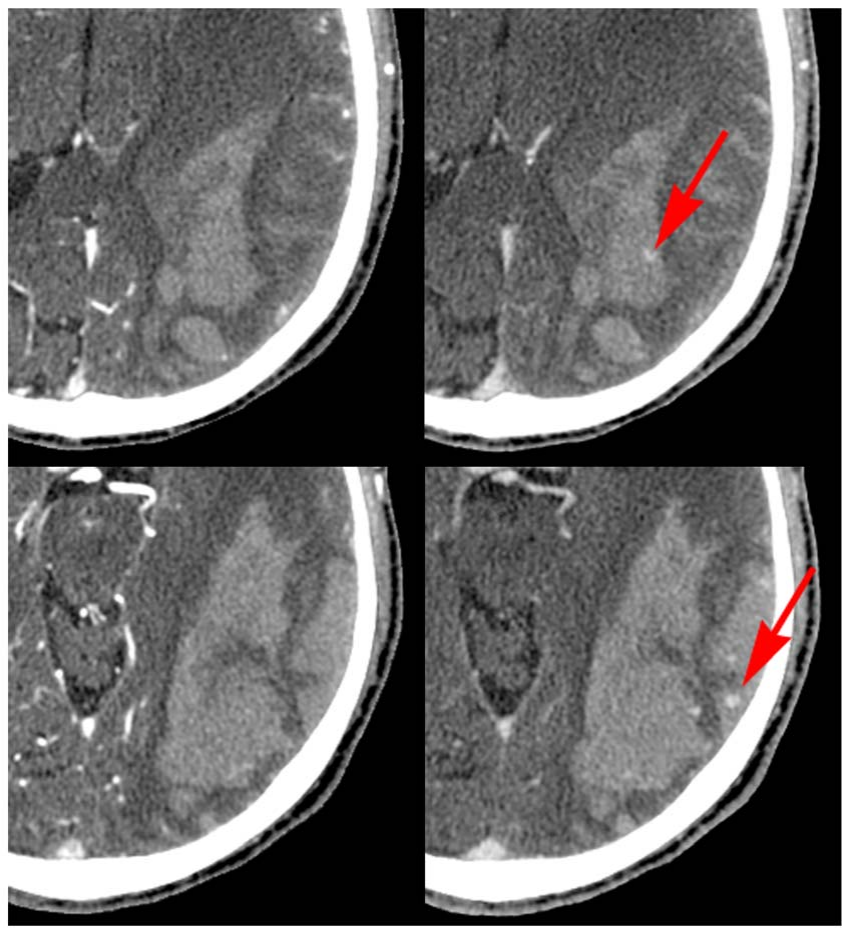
Example of active bleeding into a hematoma. 88 year old male with hypertension and acute stroke-like symptoms. CTA with delayed phase imaging shows a large hematoma in a left temporal/parietal location. Two axial levels are presented; on the left, images are from the initial phase imaging, on the right from the delayed phase. The red arrows indicate development of active extravasation seen only on the delayed views. The size of the hematoma was much larger than 80 ml and surgery was not performed, the presence of active bleeding did not change this patient's management decisions.
